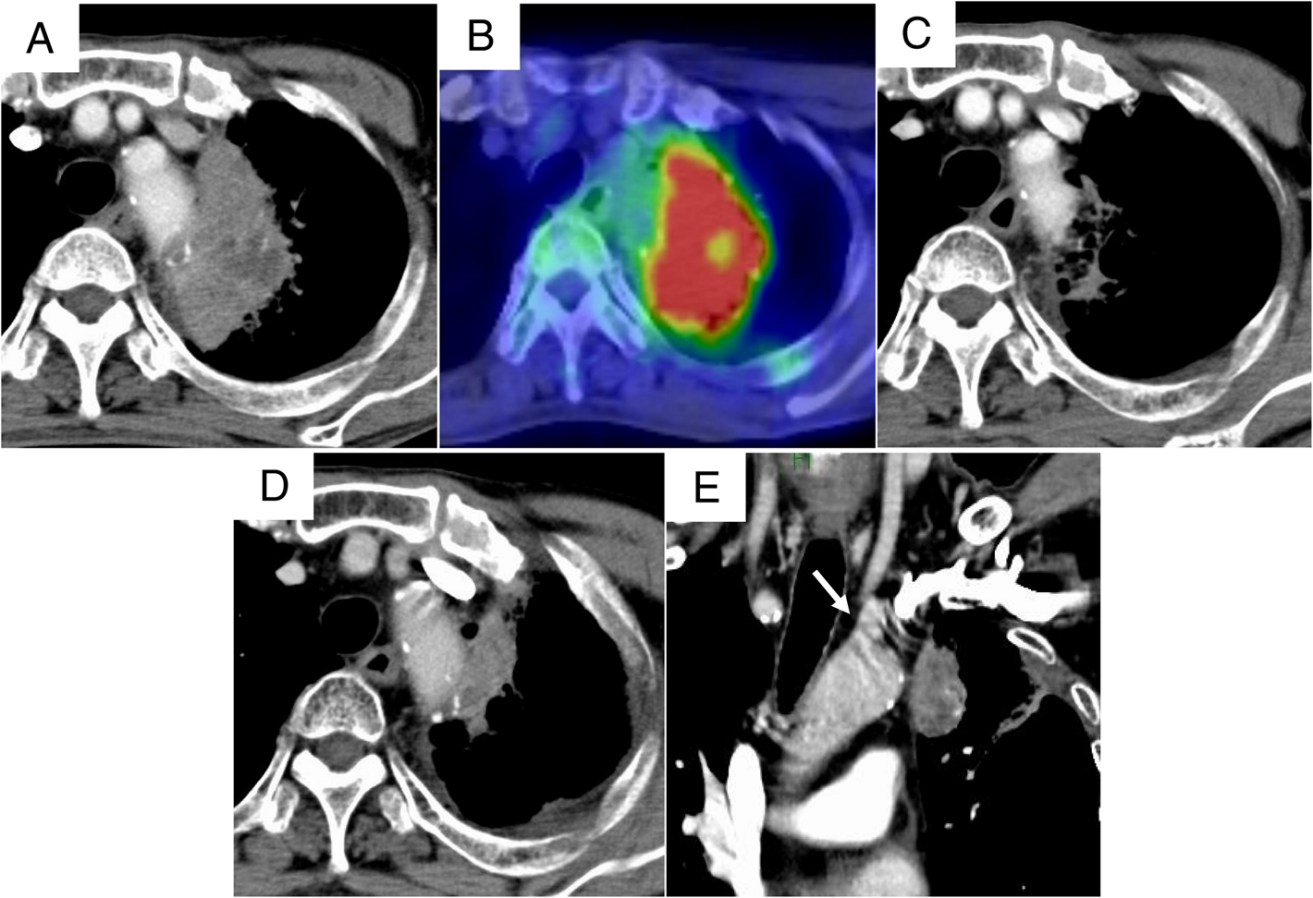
Chest contrast-enhanced computed tomography (CT) and positron emission tomography (PET)/CT findings. a, b A pulmonary tumor originated from the left upper lobe suspected to have invaded the aorta. c Markedly reduced tumor size following definitive chemo-radiotherapy. d The re-enlargement of primary tumor during steroid tapering. e Aortic invasion localized in the periphery of the left subclavian artery (arrow)
